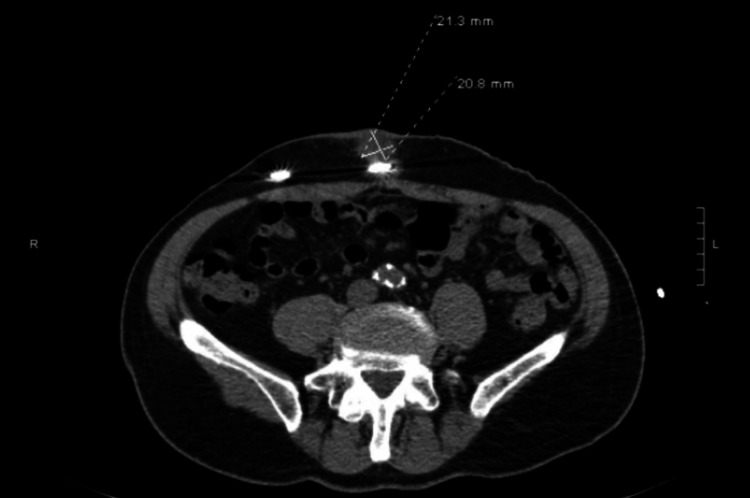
CT of abdomen and pelvis without contrast demonstrating a 2.1 x 2.0 cm sized fluid collection along the subcutaneous aspect of the drive line in the infra-umbilical region.
